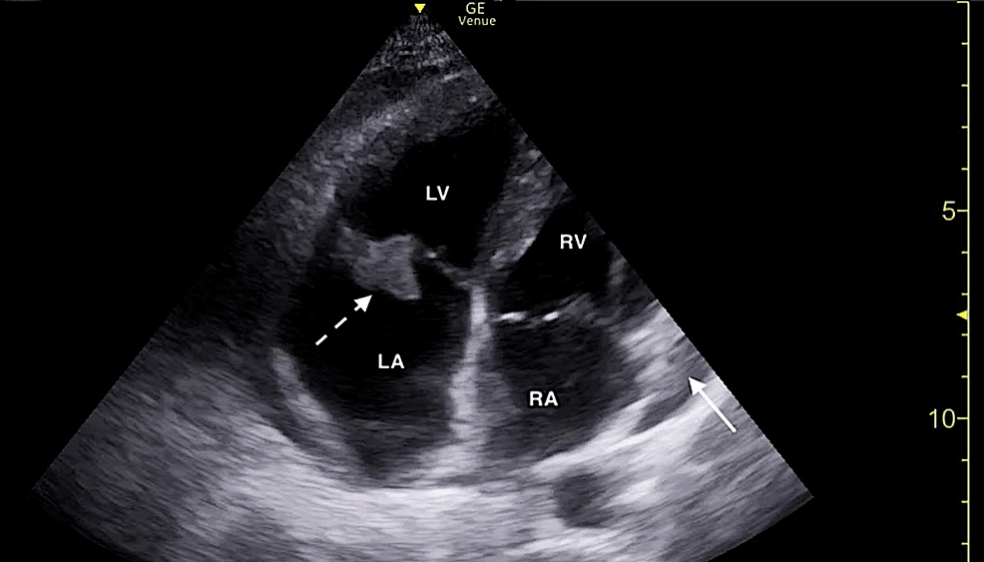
Focused cardiac ultrasound demonstrating a tricuspid valve vegetation (dotted white arrow) and small pericardial effusion (solid white arrow) in the apical four-chamber window. RA, right atrium; RV, right ventricle; LV, left ventricle; LA, left atrium
